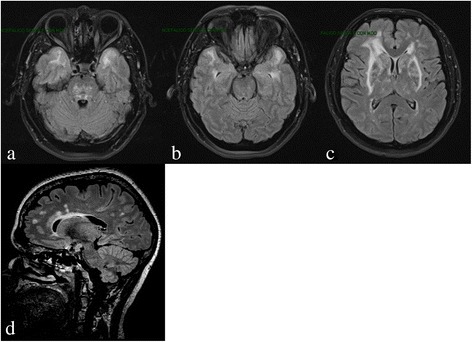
Axial FLAIR MRI: Multifocal/confluent subcortical white matter lesions, with involvement of anterior temporal lobes (a, b), pons (a), external capsules, periventricular and fronto-pariental regions (c), and corpus callosum (d)
